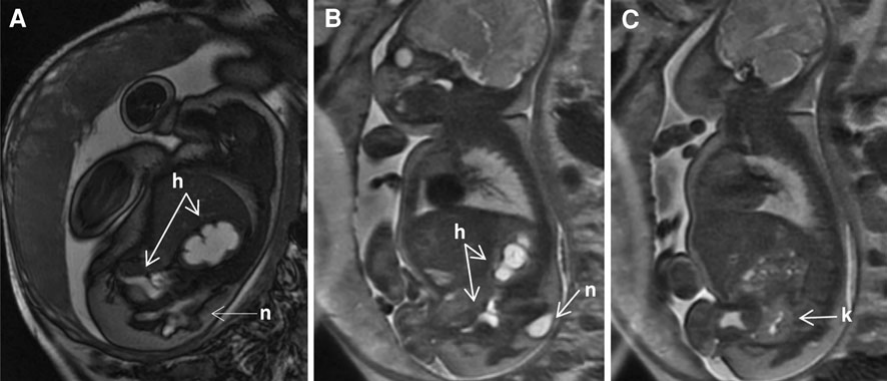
Prenatal MRI of a patient with cloacal exstrophy showing severe hydronephrosis and hydroureter (*h*) (a), neural tube defect (*n*) (a, b), and a pelvic kidney = k (c)
